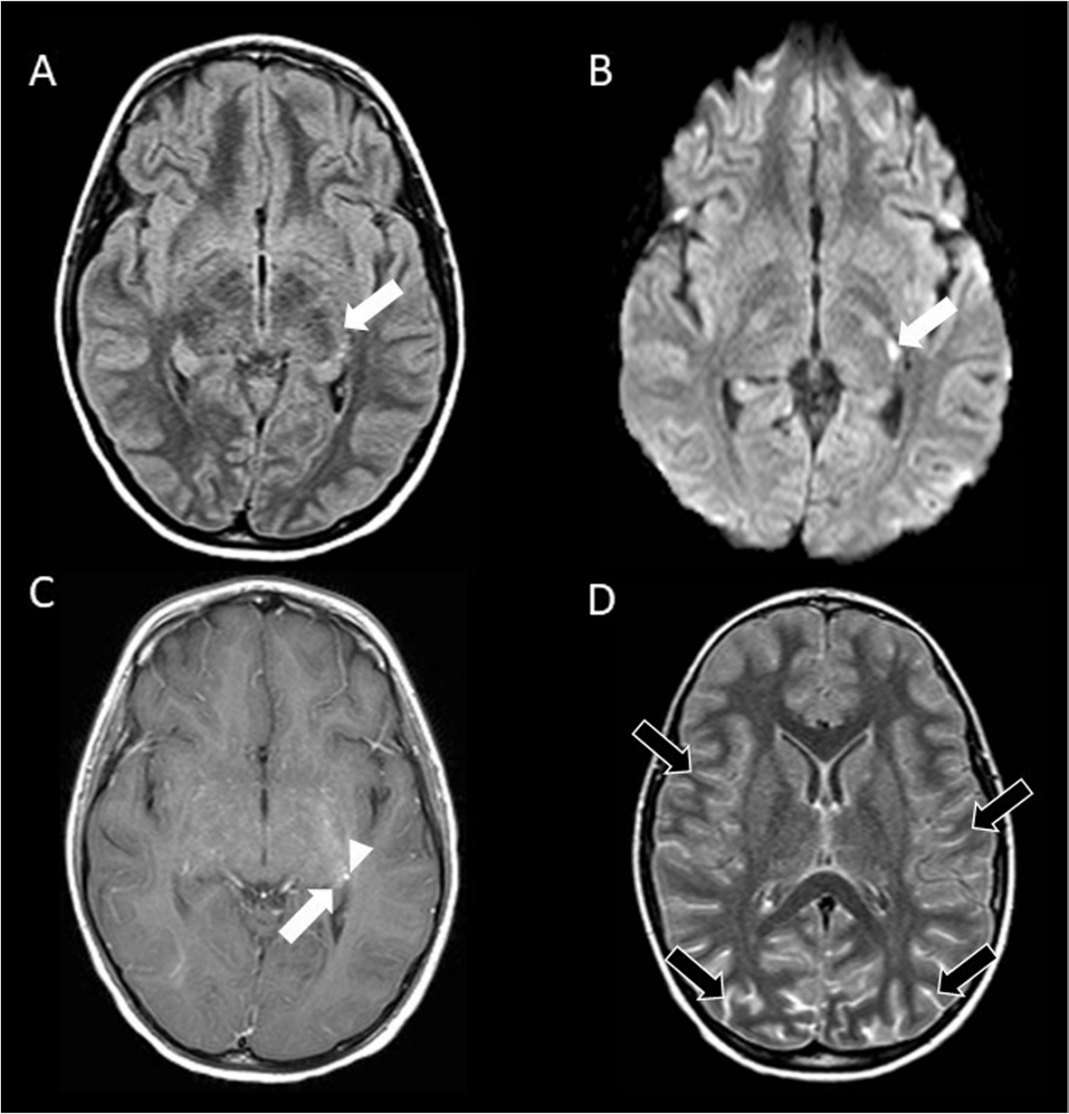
MRI of the brain obtained on day 5 of admission. A-C. Axial pre-contrast FLAIR (A), DWI (B) and post-contrast T1-weighted (C) MRI demonstrate a small FLAIR hyperintense lesion (white arrows) with low diffusivity and faint enhancement in the left thalamus (noting incidental adjacent developmental venous anomaly, arrowhead in C). D. Axial postcontrast FLAIR demonstrates diffuse leptomeningeal enhancement (black arrows) that was not apparent on the post-contrast T1-weighted images
